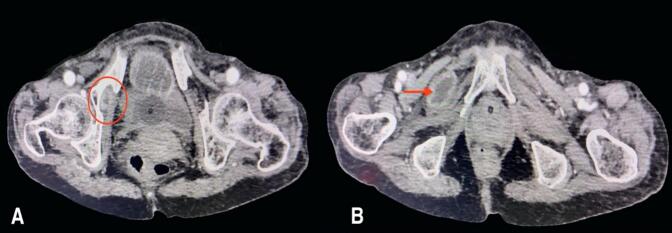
CECT Axial section- A: Small bowel herniating through the right obturator canal. B: Small bowel loop trapped between obturator externus and pectineus muscle. CECT: Contrast-enhanced computed tomography.
